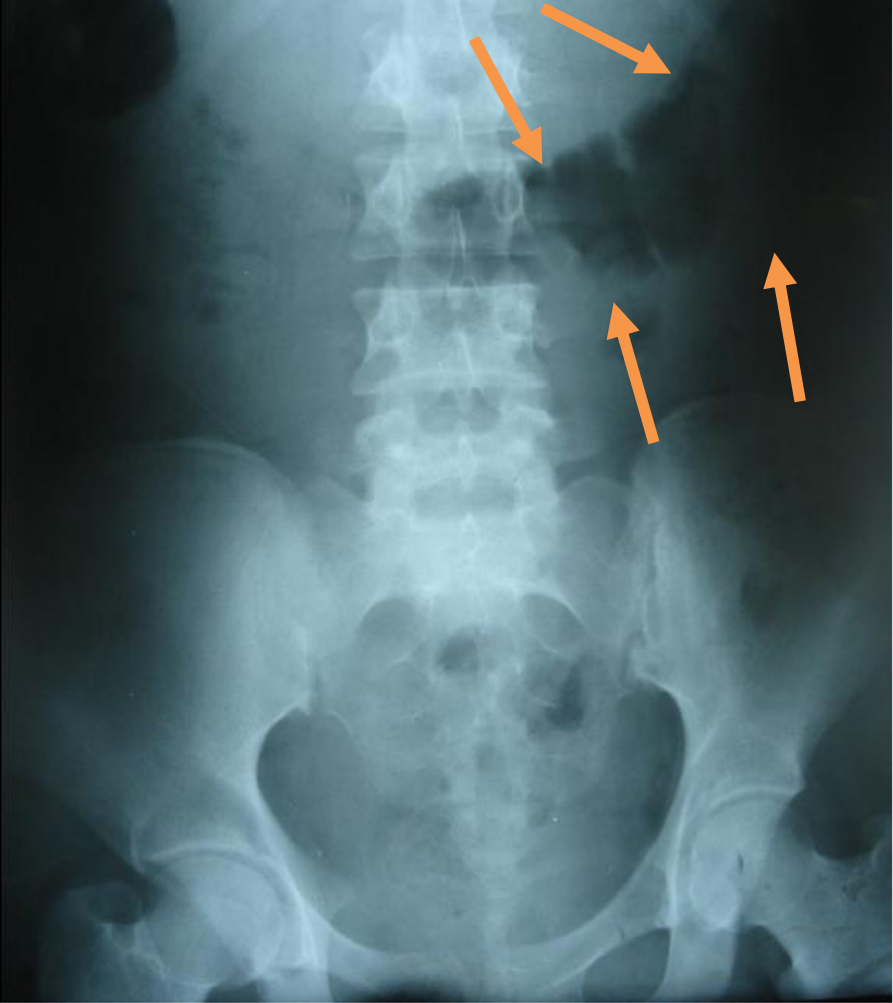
Plain X-ray of the abdomen showing a well-defined, rounded soft tissue density mass, in the central abdominal region with calcification (arrows).
